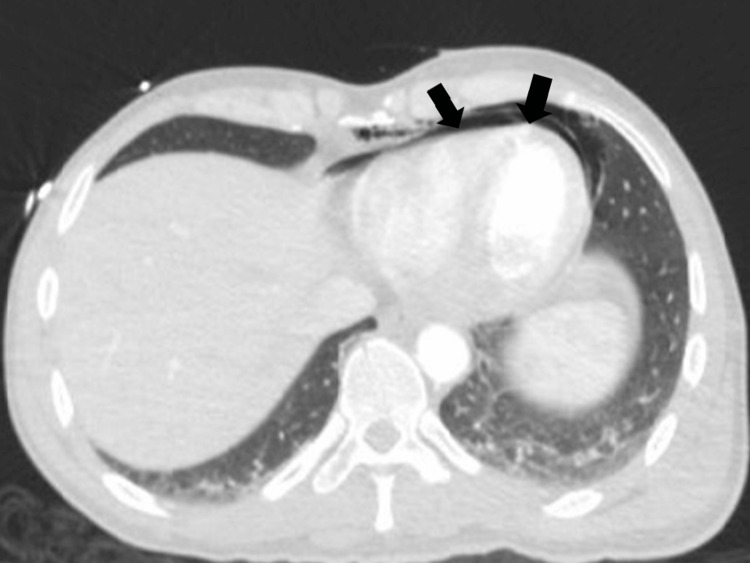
Admission chest computed tomography confirming the pneumopericardium.
